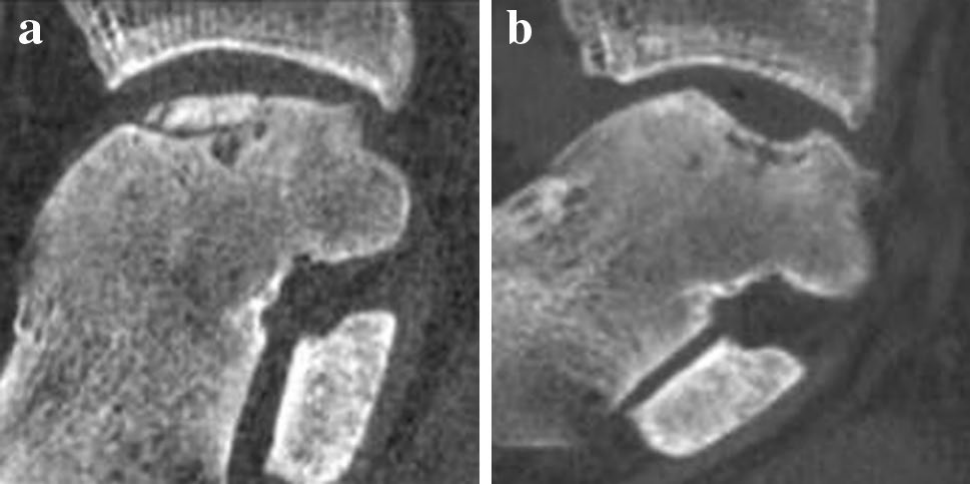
a Preoperative sagittal CT of a medial osteochondral talar defect of a right ankle. b Postoperative sagittal CT of the same ankle after arthroscopic debridement and bone marrow stimulation (BMS) at 1-year follow-up
